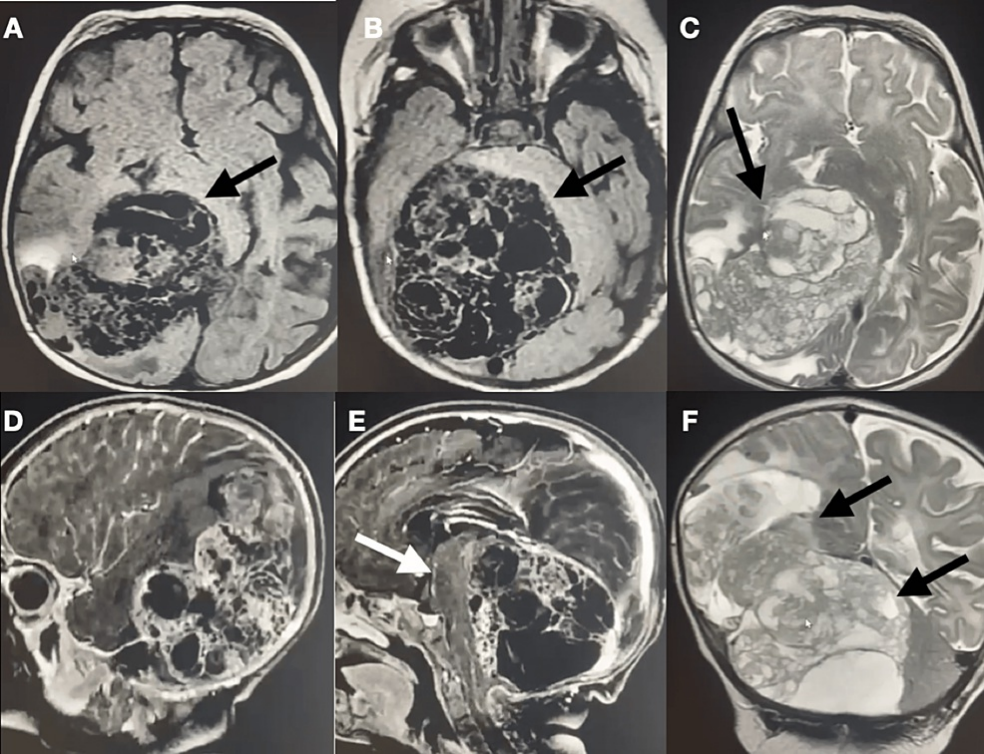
Magnetic Resonance Imaging (A and B) Axial T1, large heterogeneous lesion with significant mass effect with brain stem compression (black arrow); (C) Axial T2, massive infiltrative lesion (black arrow); (D and E) Sagittal view with contrast, tumor compressing the brainstem(white arrow); (F) Coronal T2, tumor in the supra and infratentorial compartment (black arrows)
